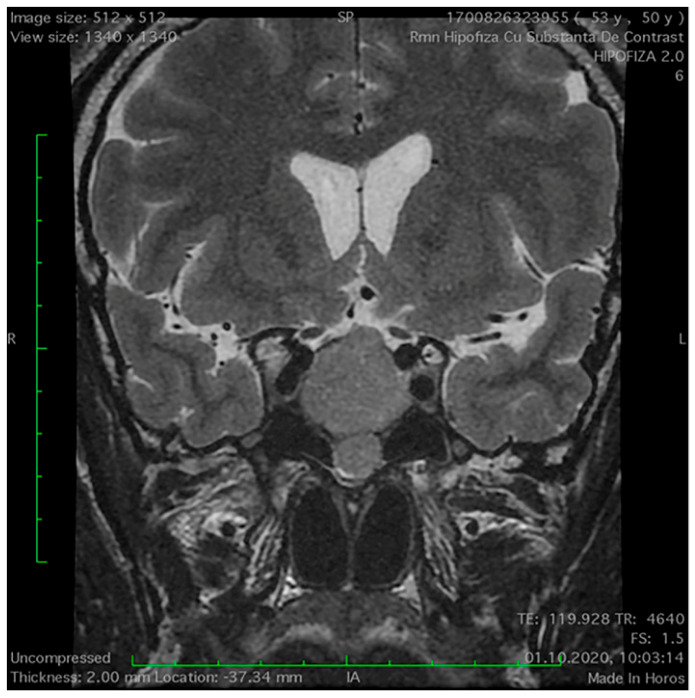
Measurement of the distance between the two optic nerves on a coronal MRI section.
